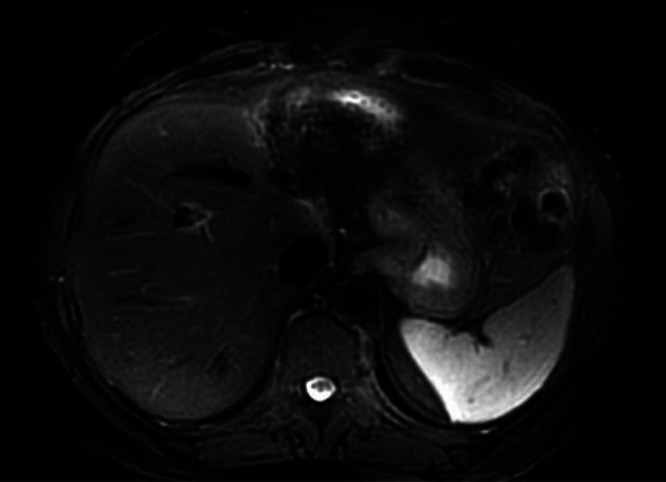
One month after treatment, magnetic resonance imaging shows disappear of pseudotumoral lesions on liver.
